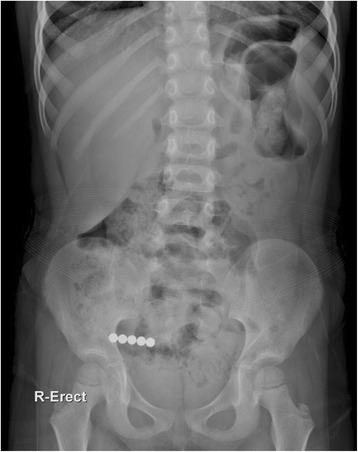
Abdomen radiography showed beaded foreign material on right lower quadrant
